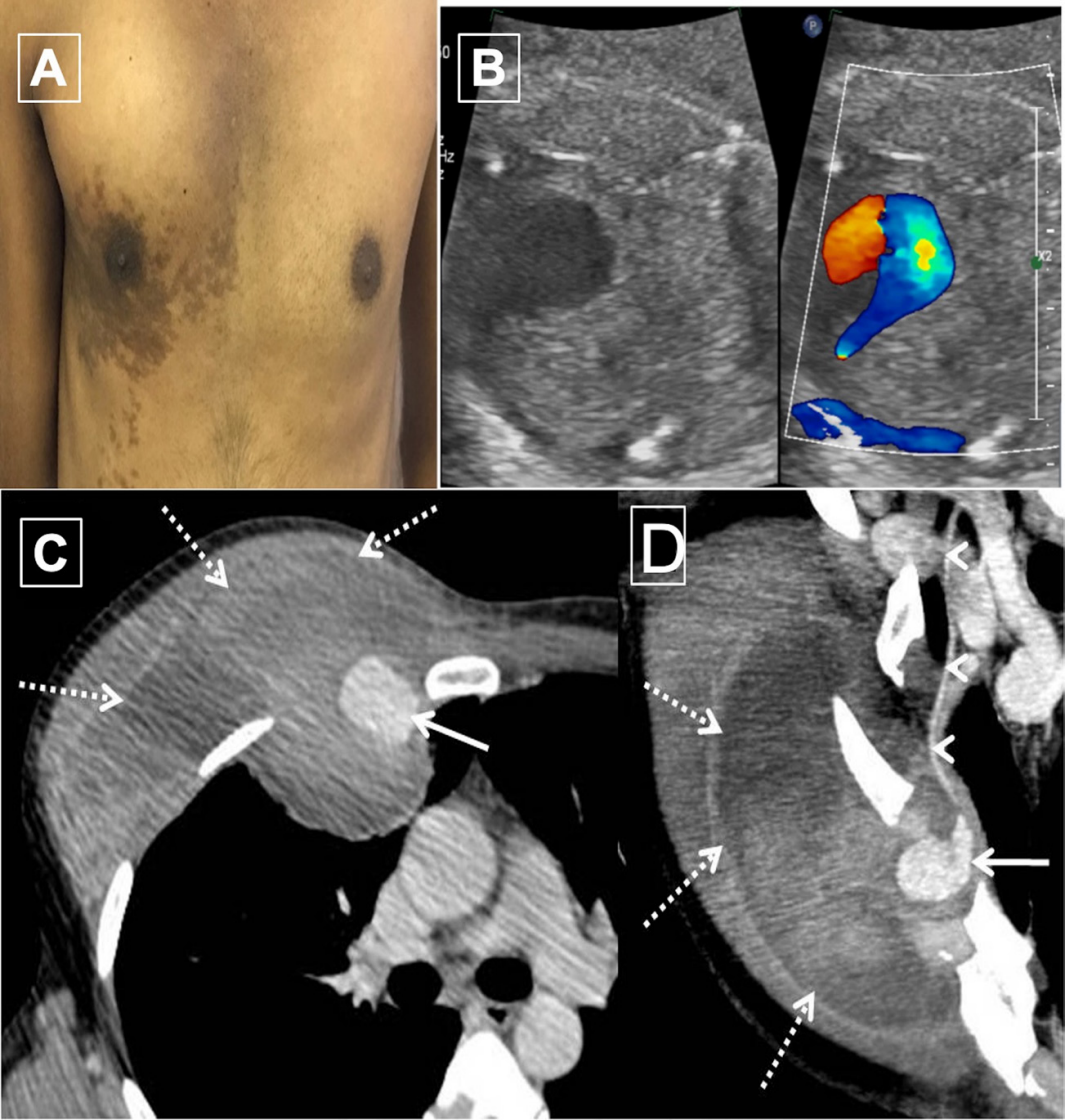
(A) Clinical photograph showing bulge in the right mammary and infraclavicular areas; (B) Right internal thoracic artery (RITA) aneurysm showing bidirectional blood flow with characteristic yin yang sign; (C and D) Pre-coiling CT: CT angiography images show presence of ruptured aneurysm sac (arrow) arising from the RITA (arrowheads) with surrounding large hematoma (dashed arrows) causing contour bulge along anterior chest wall on right side.
